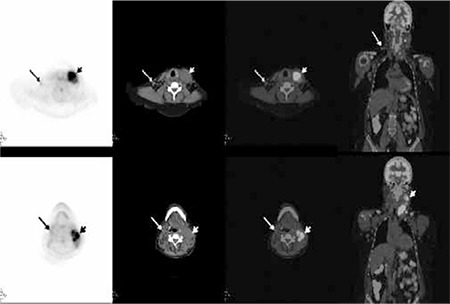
Axial PET, CT, PET/CT fusion and coronal PET/CT fusion images revealed left submandibular and upper-middle jugular conglomerate lymph nodes (short arrow) with intense FDG uptake (SUVmax was 13.0) suggesting transformation. Additionally, minimally increased FDG uptake in the right jugular chain (long arrow) compatible with indolent lymphoma involvement was noted.
